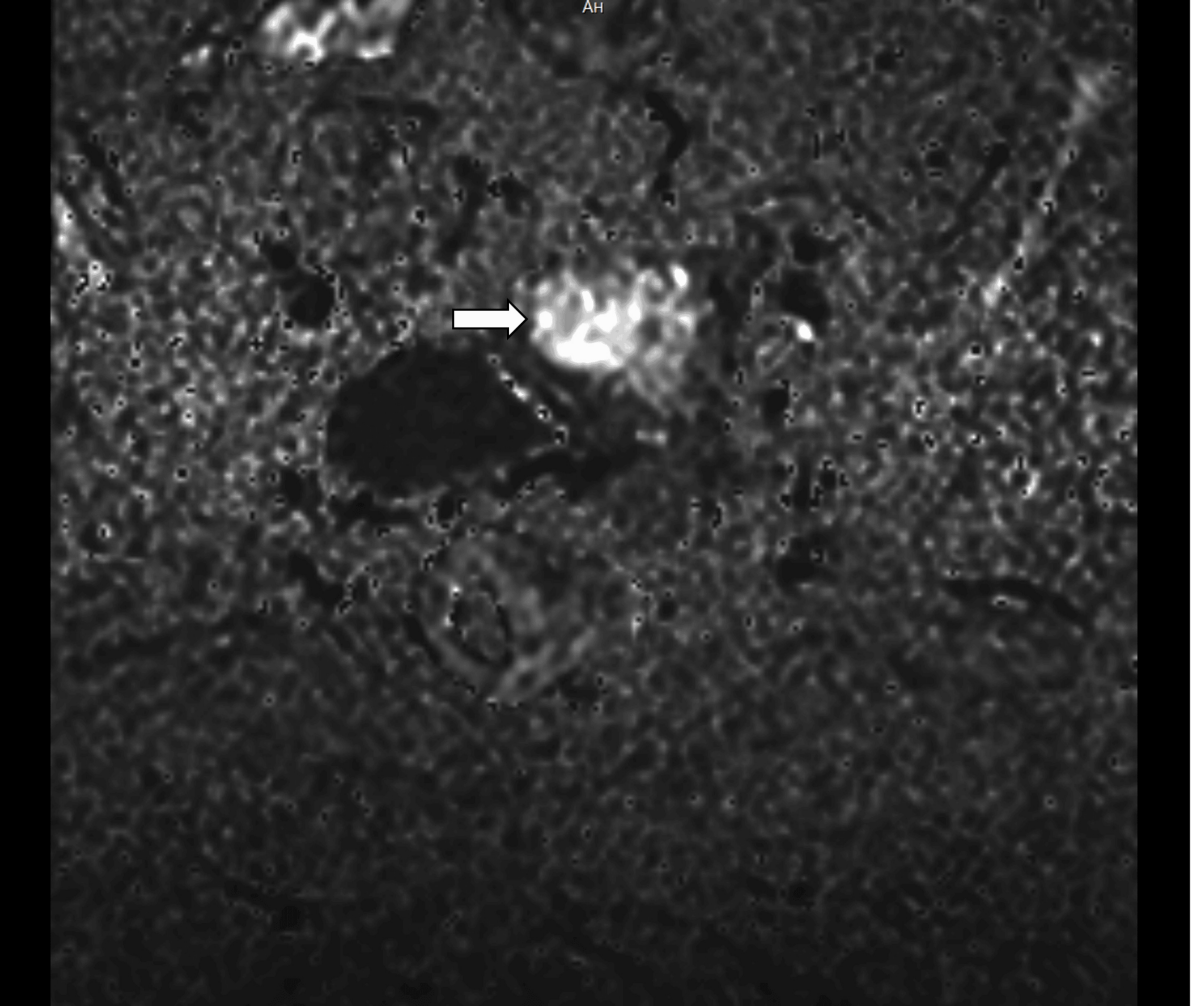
Diffusion-weighted imaging (DWI) with a b-value of 2000 s/mm² demonstrates marked diffusion restriction within the endometrial lesion (arrows), raising suspicion for malignancy. b-value – Diffusion sensitivity factor used in diffusion-weighted MRI
